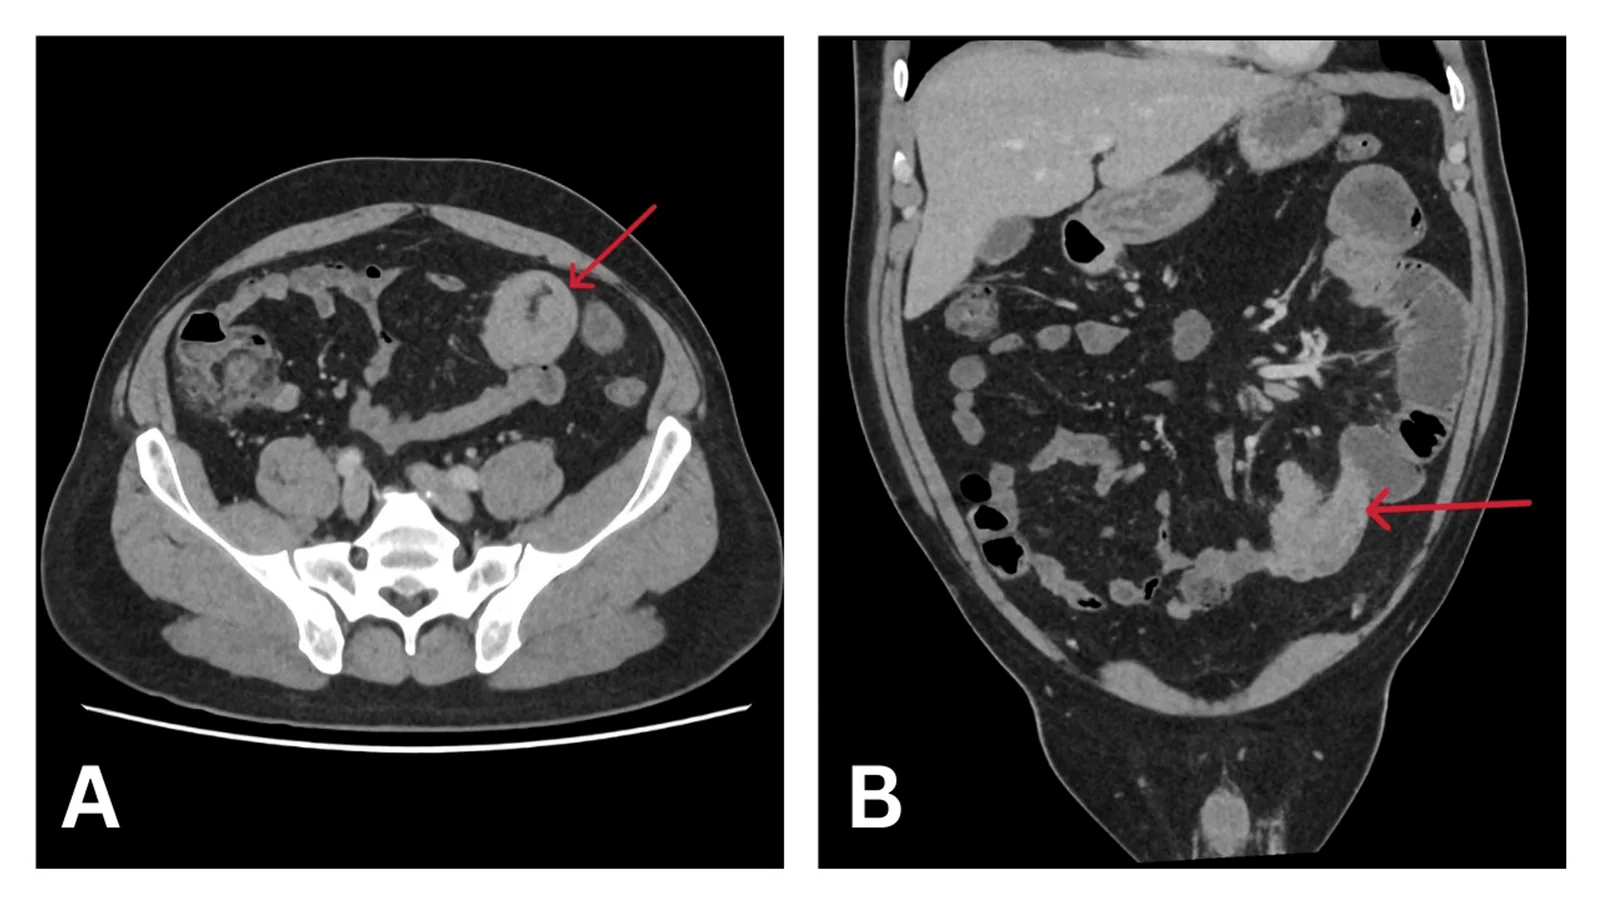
A: Axial CT image demonstrating a well-defined soft tissue mass (arrow) with a central mesenteric fat/vessel component, in keeping with a target sign of small bowel intussusception. B: Coronal oblique reconstruction demonstrating the bowel within the bowel sign of small bowel intussusception (arrow), corresponding to the longitudinal appearance of the intussuscepted bowel segment.
